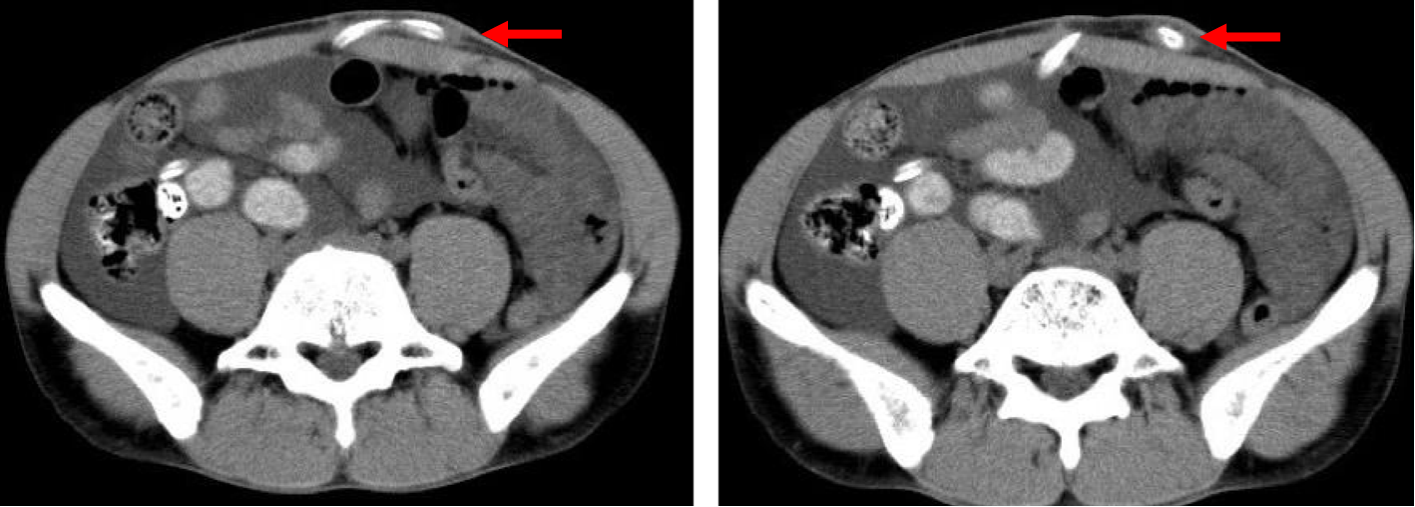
Images of computed tomography. Increased density observed around the peritoneal dialysis catheter (arrows)
